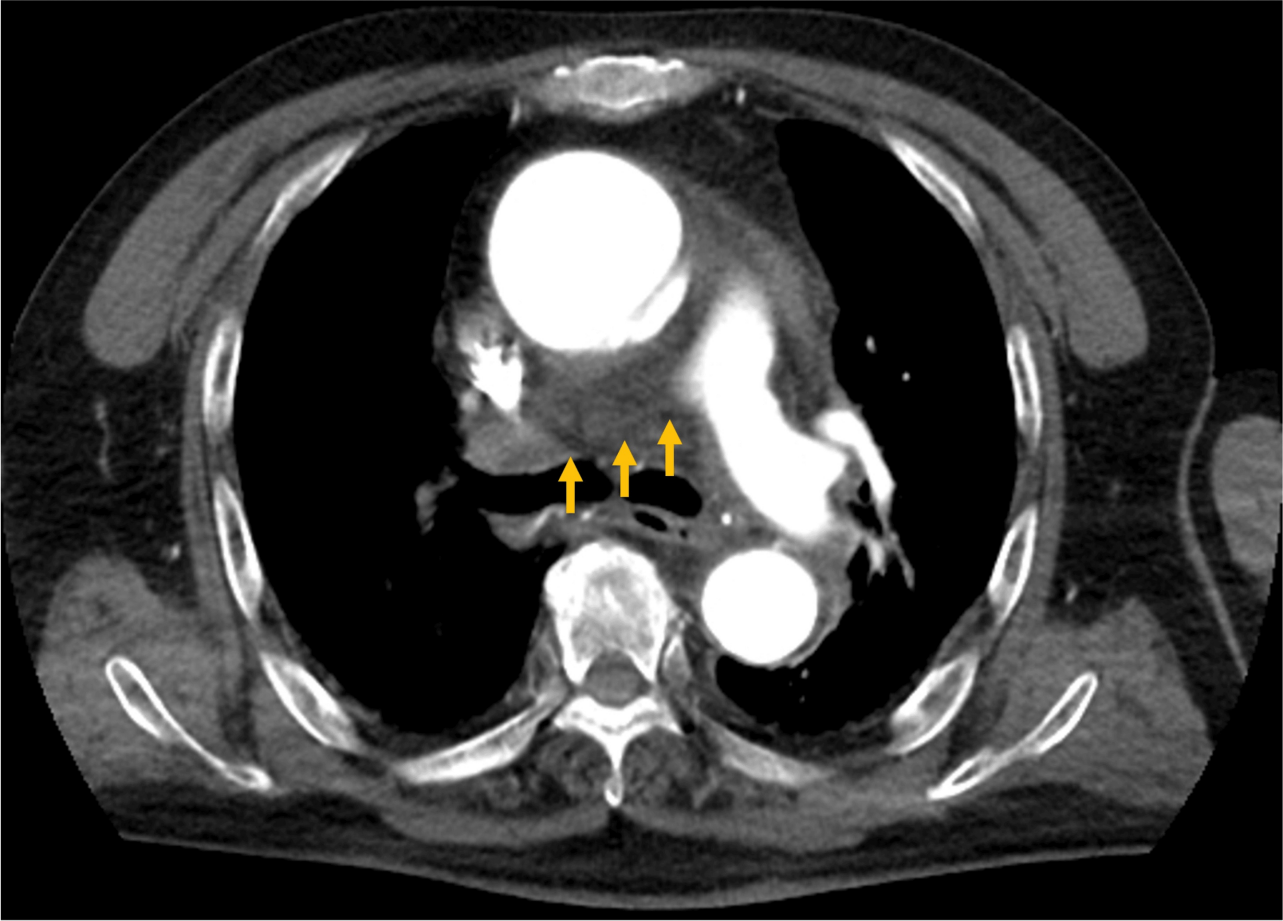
Contrast-enhanced computed tomography showing occlusion of the right pulmonary artery just distal to its bifurcation from the main pulmonary artery (yellow arrow)
